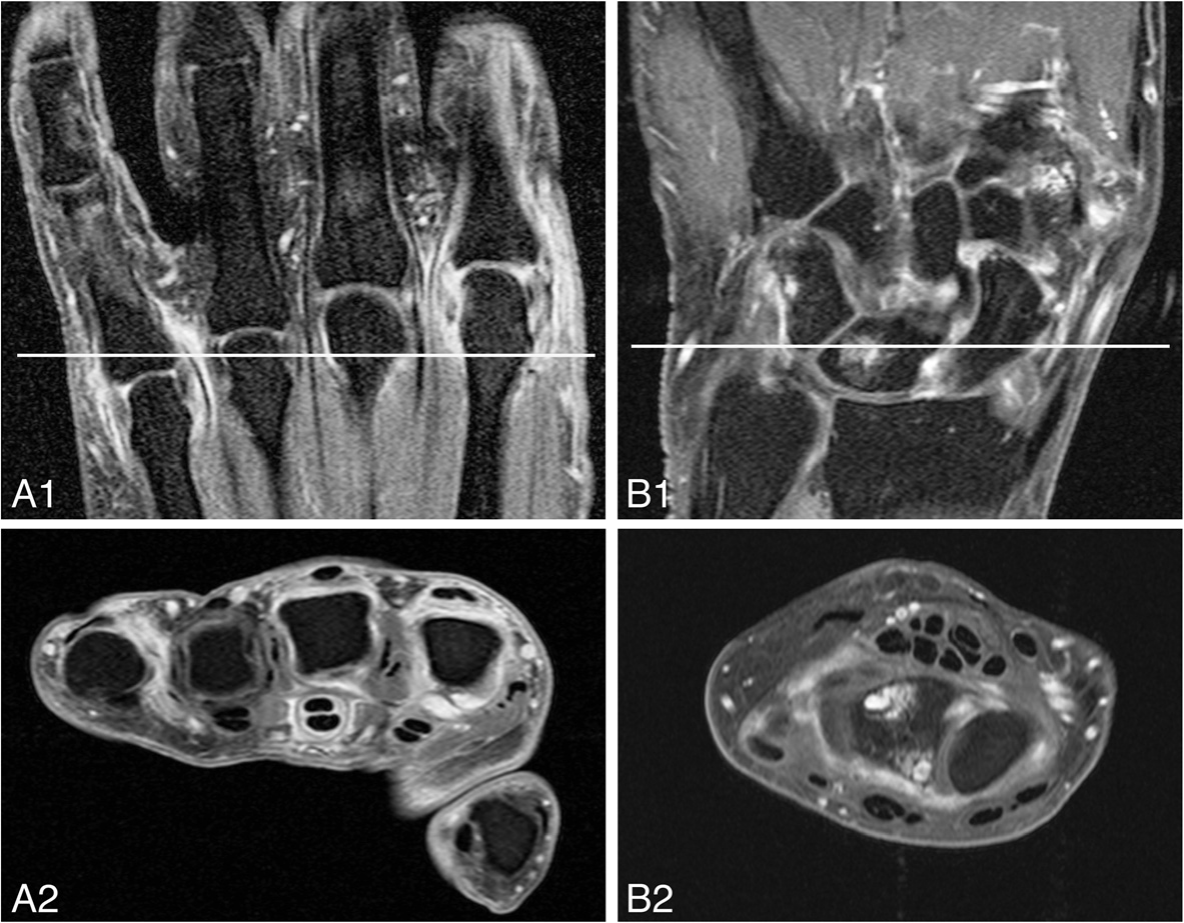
Subclinical inflammation visualised by magnetic resonance imaging of two different anticitrullinated peptide antibody–negative arthralgia patients without clinically detectable arthritis. Images show the metacarpophalangeal (MCP) joints and wrists of anticitrullinated peptide antibody (ACPA)–negative arthralgia patients without clinically detectable arthritis. The white lines in the top coronal images reflect the localisation of the bottom axial images. (A) Post–contrast enhancement coronal (A1) and axial (A2) T1-weighted fast spin echo (FSE) images with fat saturation showing enhancement of the MCP2, MCP3 and MCP5 joints, which is consistent with active synovitis. Also, pronounced tenosynovitis in the third flexor tendon is present, although tenosynovitis is not included in the OMERACT rheumatoid arthritis magnetic resonance imaging scoring system score and was not evaluated in the present study. This patient developed clinically detectable arthritis during follow-up. (B) Post–contrast enhancement coronal (B1) and axial (B2) T1-weighted FSE images with fat saturation showing bone marrow oedema (BME) and erosions (confirmed on the pre–contrast enhancement T1-weighted FSE sequence) in the lunate. Also, there is active synovitis in the intercarpal joint.
